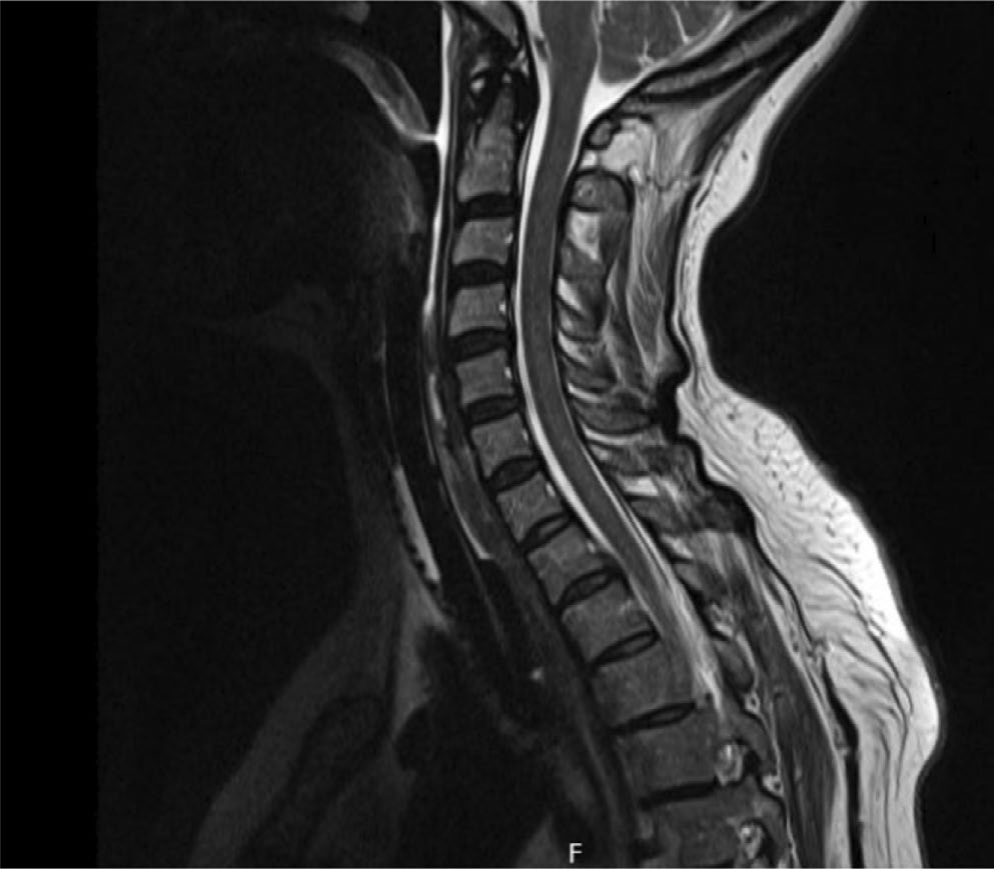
FIGURE 2 Spinal cord lesions in MRI T2 sequence
